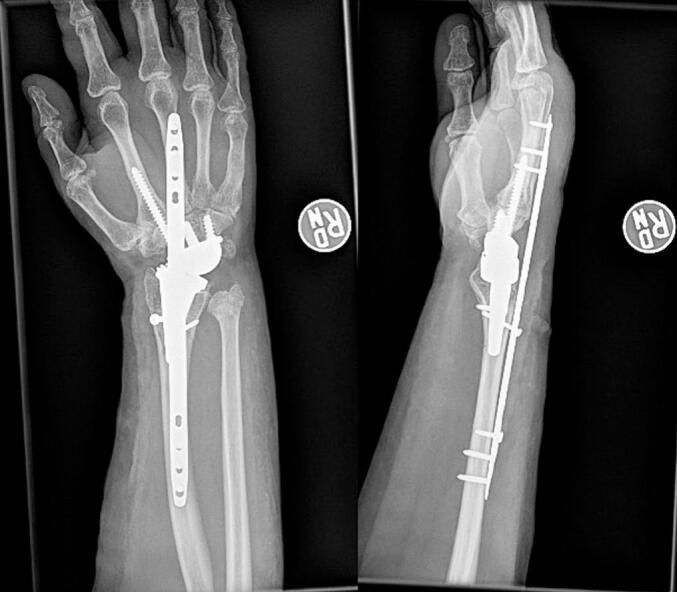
Right wrist radiograph 1 week post-operatively.
